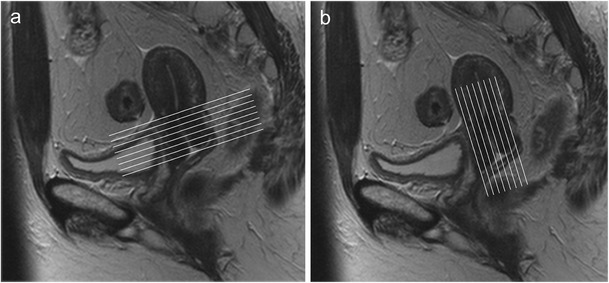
Sagittal T2-weighted image. The oblique axial (a) and coronal planes (b) are orientated to the plane of the cervical canal
